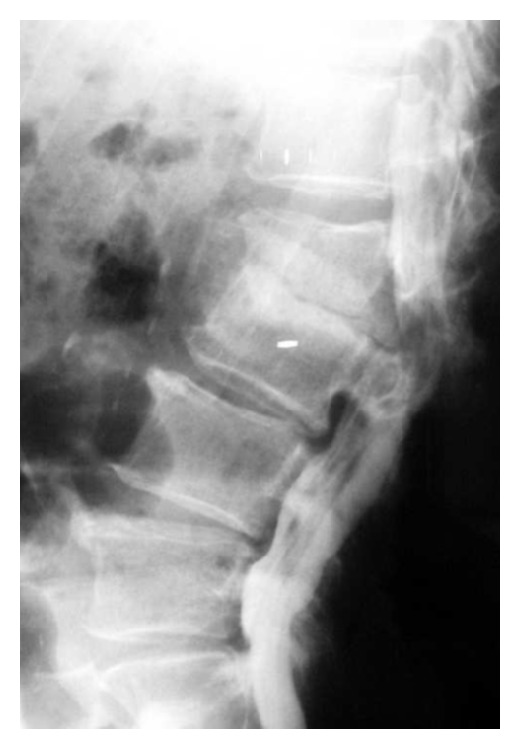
Preoperative plain radiograph (lateral view) showing marked narrowing of L2-L3 disc space with some endplate destruction; new bone formation is noted (Case 1).
